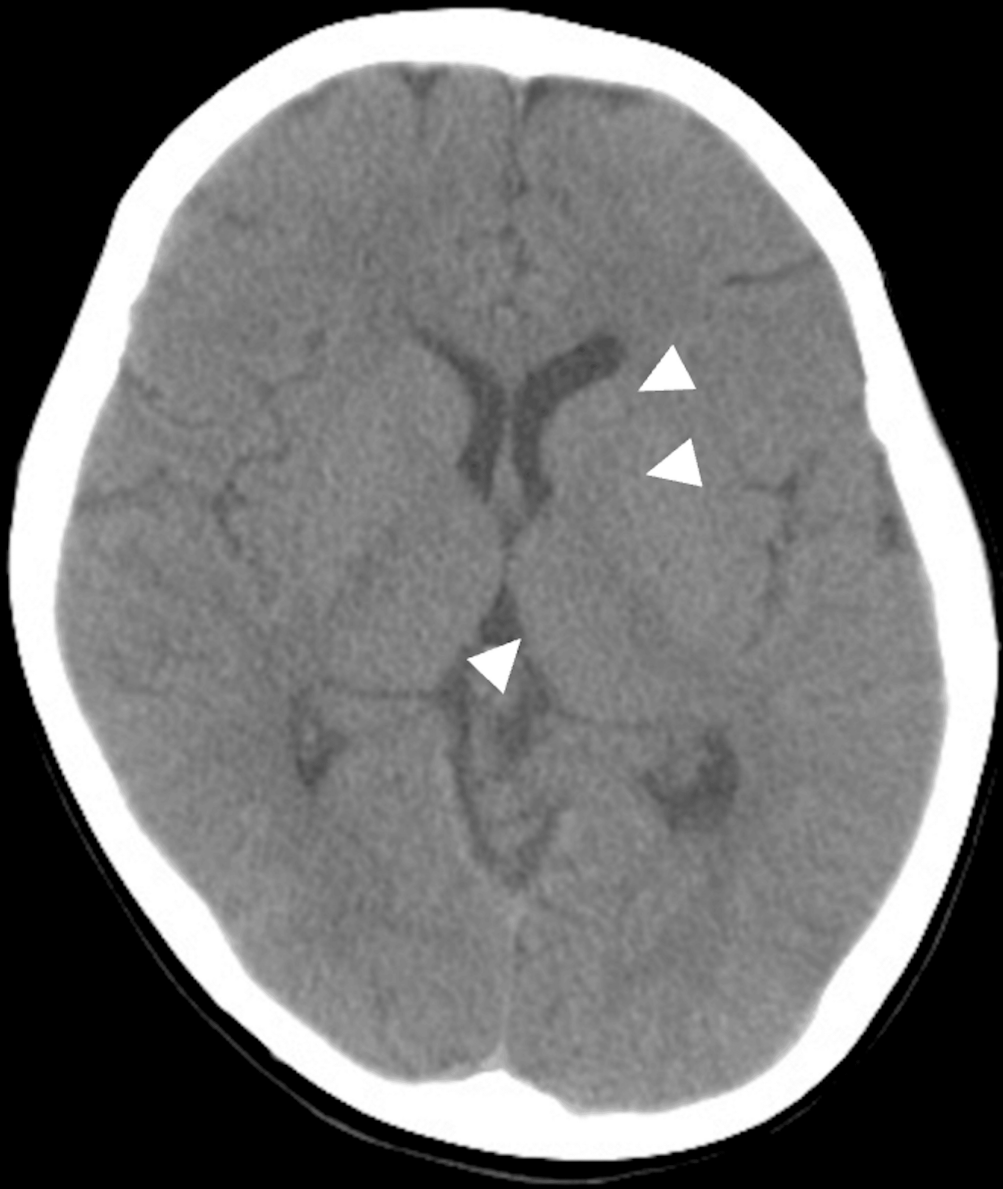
Non-contrast head CT at age 6 A non-contrast head CT shows no calcifications or structural abnormalities. White arrowheads indicate the basal ganglia and thalamus as regions of interest, where no abnormalities are present at this age. This normal scan serves as a baseline for comparison with later imaging.
